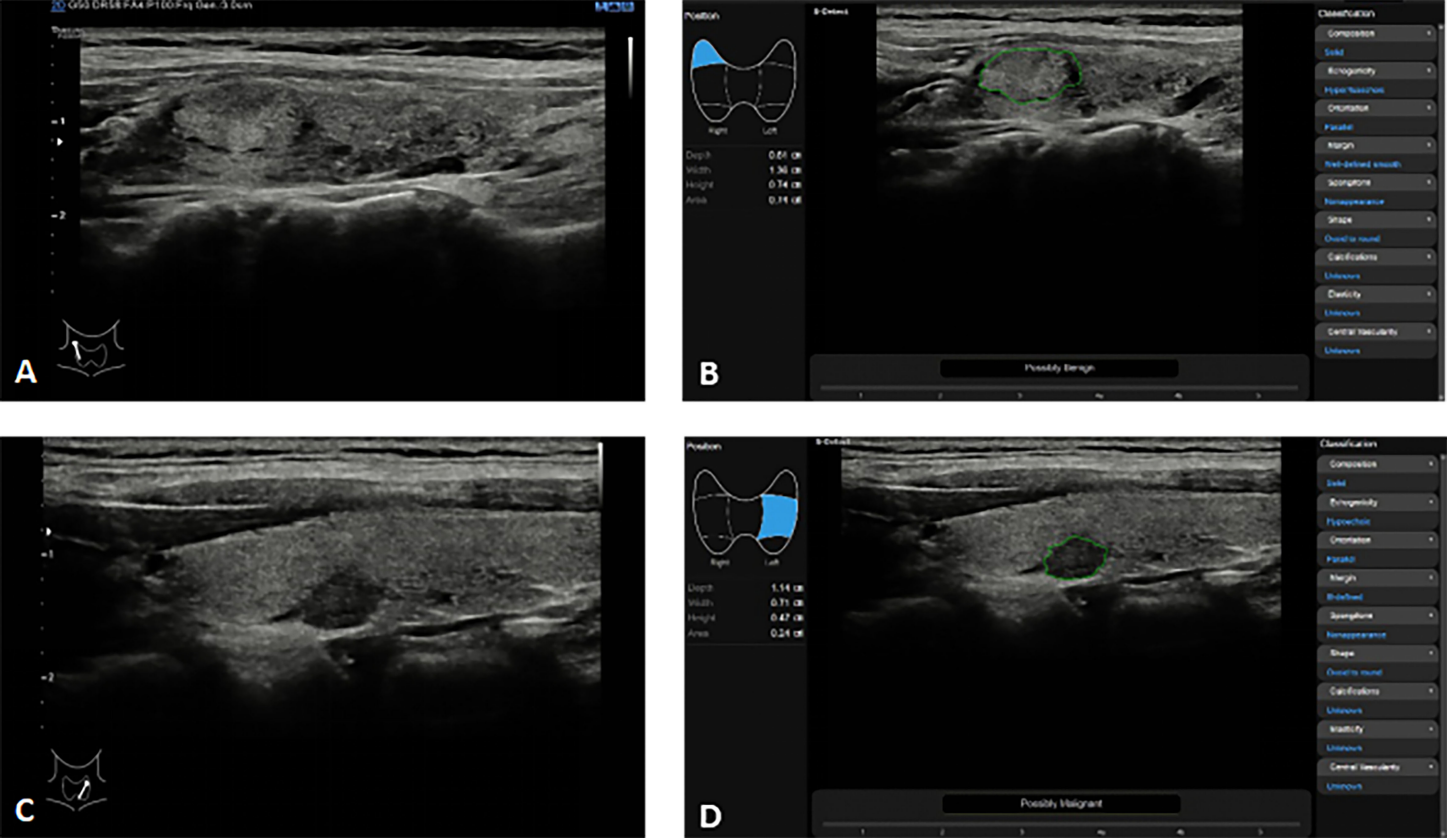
Thyroid nodules S-detect technique in the Samsung RS80A ultrasound system. (A, B) In a 35-year-old woman with right Hashimoto’s thyroiditis with focal fibrosis on conventional ultrasound (A), S-Detect comes to the correct conclusion through analysis as “Possibly Benign” (B); (C, D) In a 52-year-old woman with left thyroid cancer on conventional ultrasound (C), S-Detect comes to the correct conclusion through analysis as “Possibly Malignant” (D).
